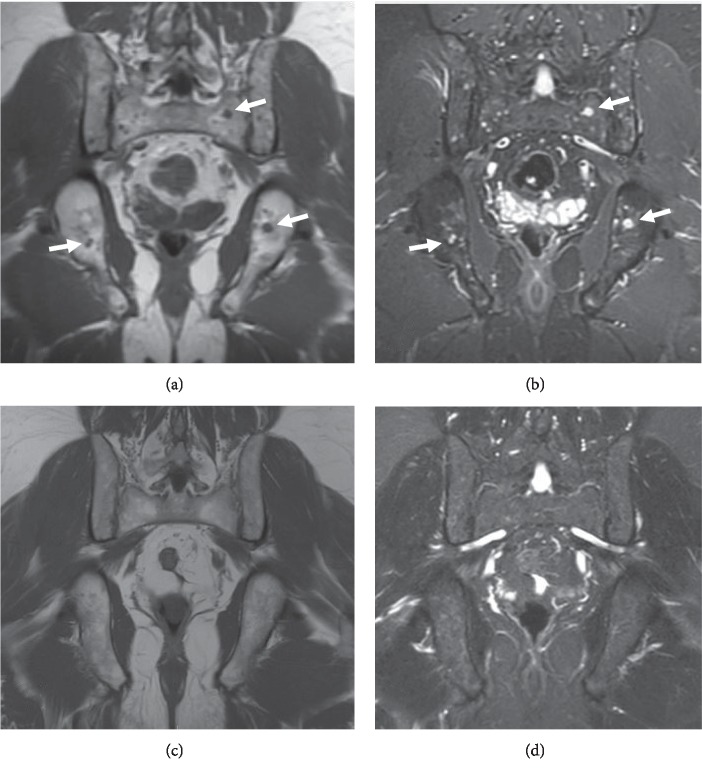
T1-weighted (a, c) and STIR (b, d) coronal MR images of the pelvis prior to treatment (top row) and following treatment (bottom row) show numerous small intramedullary foci of low signal on T1 and high signal on STIR (representative arrows), which essentially resolve following treatment.
